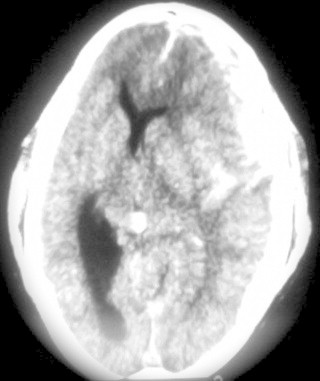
CT scan on admission showing extensive acute left subdural hematoma.
